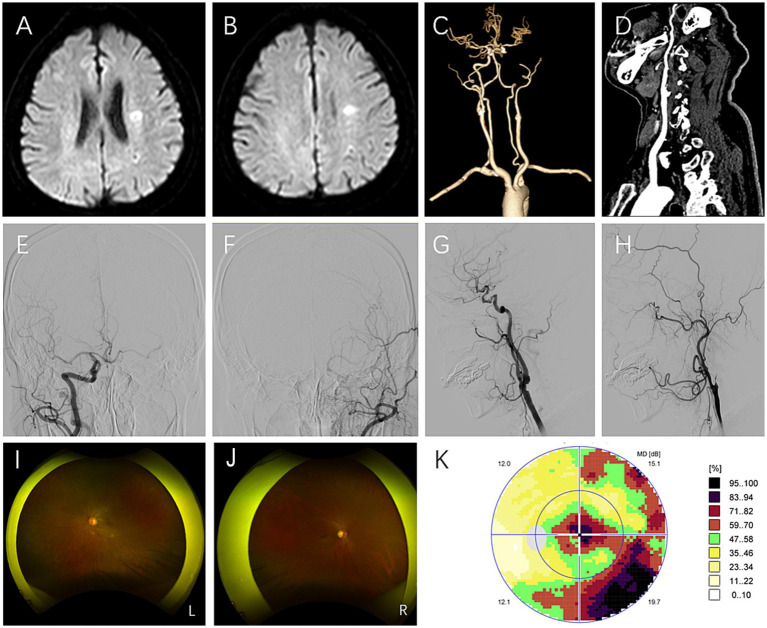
Imaging findings of Case 2. (A,B) DWI showing new infarcts in the left corona radiata and parietal lobe. (C,D) CTA showing occlusion of the left ICA origin. (E–H) DSA showing patent ICA stump with turbulent flow; distal vessel occluded. (I–K) Ophthalmic fluorescein fundus angiography showing a large avascular area in the left retina.
